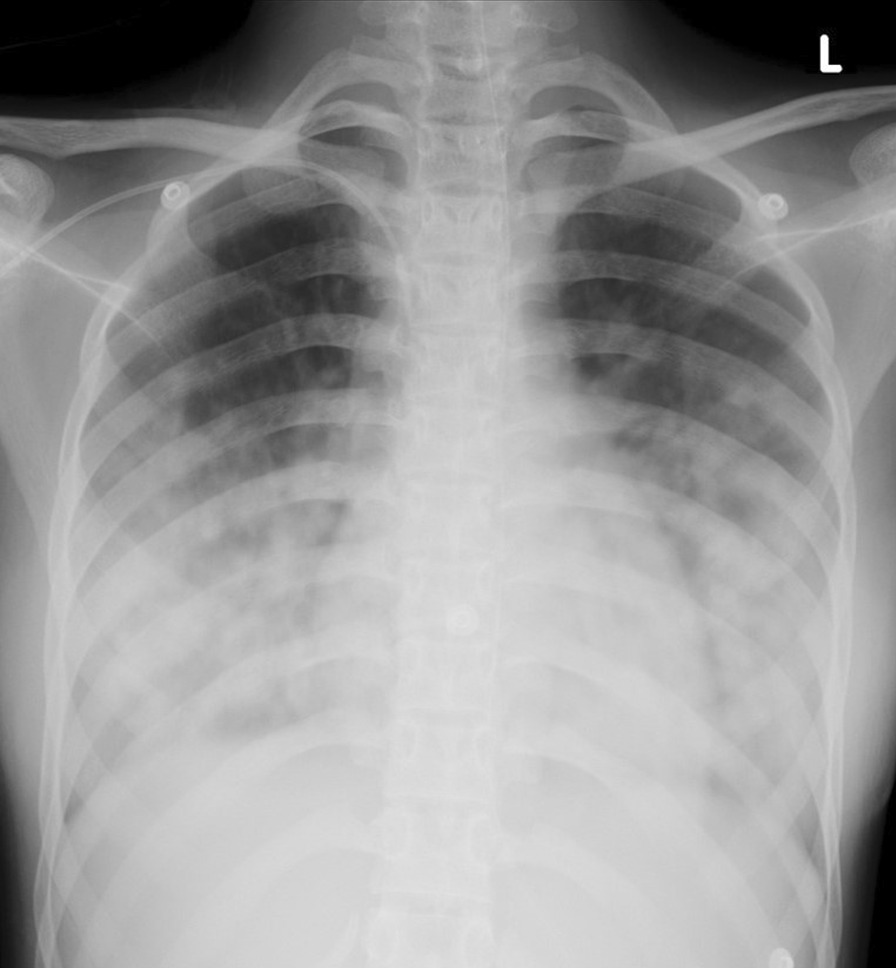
Chest radiograph showing bilateral middle and lower zone alveolar opacities with sparing of upper zones
